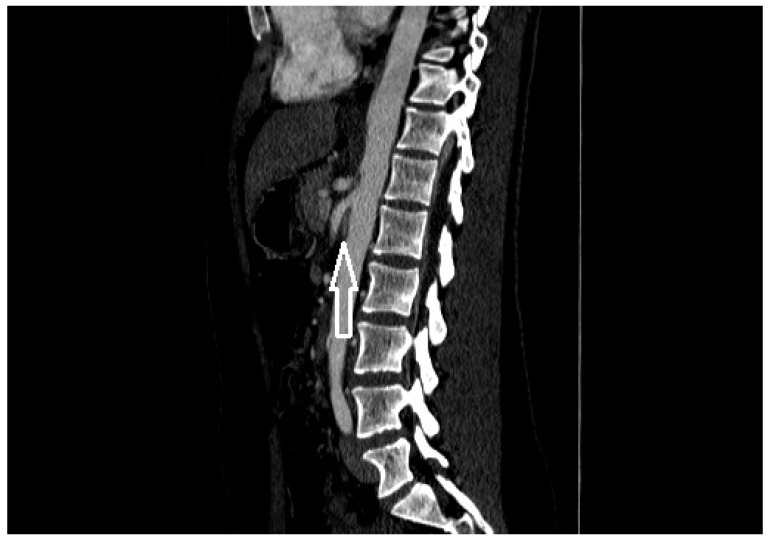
A patient with nutcracker syndrome: contrast enhanced CT scan during arterial phase, with sagittal reconstruction; in this image, it is possible to appreciate the entrapment of the left renal vein (white arrow) between the superior mesenteric artery and the aorta.
